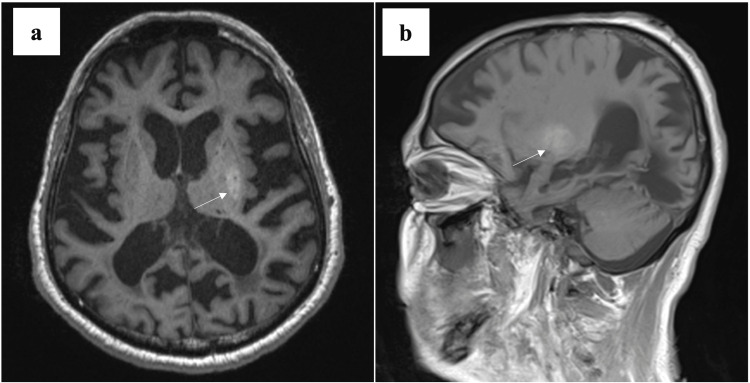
Axial (a) and sagittal (b) T1-weighted MRI demonstrating hyperintensity in the left lenticular nucleus, predominantly involving the putamen, as highlighted by arrows This finding corresponded to the left putaminal hyperdensity seen on CT and was compatible with diabetic striatopathy in the appropriate clinical context. MRI: Magnetic resonance imaging, CT: Computed tomography
